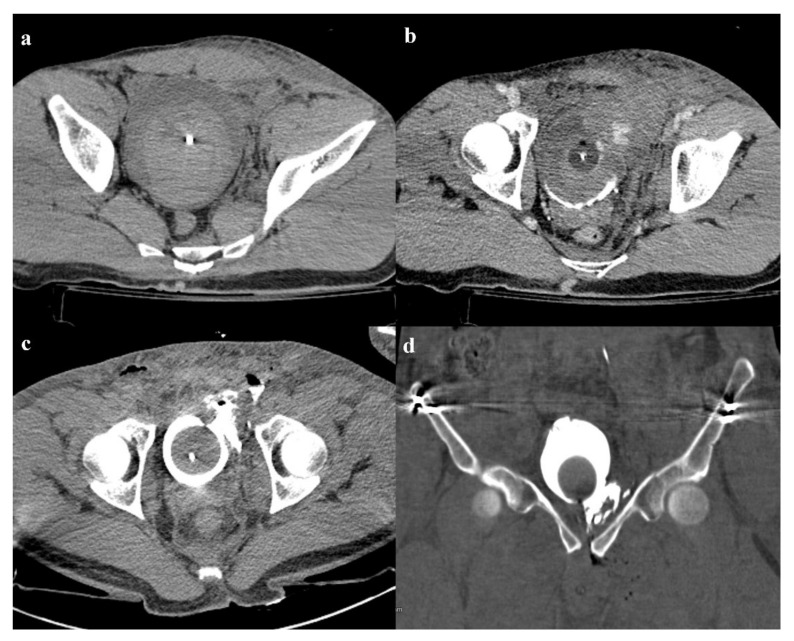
Patient of the Figure 5, with an anterior–posterior compression fracture. There is a large hematoma inside the bladder (a); contrast medium administration reveals active bleeding in the venous phase of the examination, on the left wall of the bladder (b). Axial and coronal CT cystography images show a leak of contrast medium in the perivesical space, suggestive of extraperitoneal bladder rupture (c,d).
